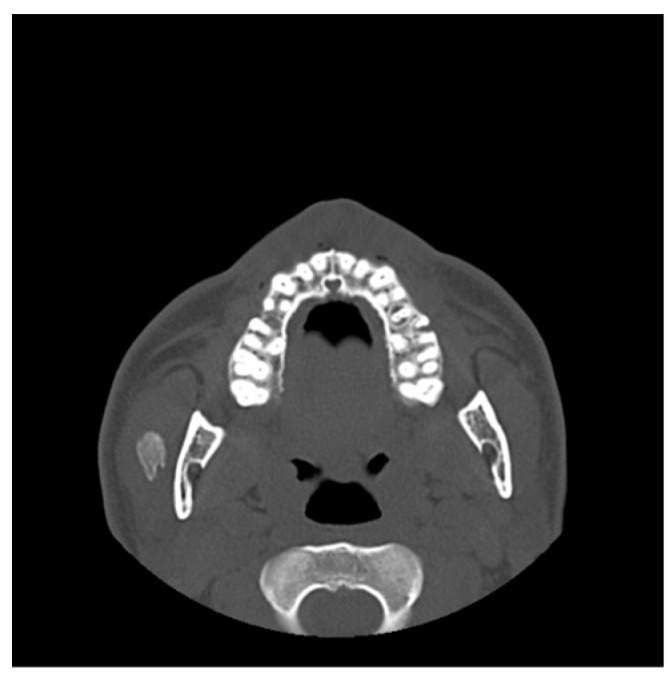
An axial CT scan showed a well-defined calcified lesion within the left masseter muscle, anatomically distinct from the previous surgical site. The radiologic appearance is consistent with recurrent or new-onset myositis ossificans.
